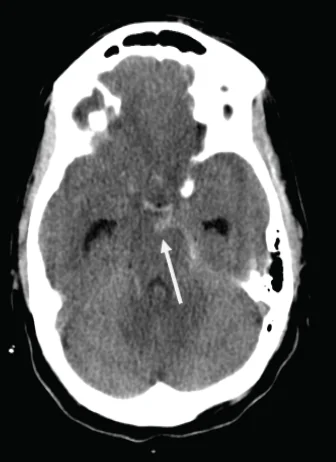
Computed tomography of the head (axial view) showing hemorrhage extension into the perimesencephalic cisterns within the prepontine cistern, slightly asymmetric to the left (arrow).
